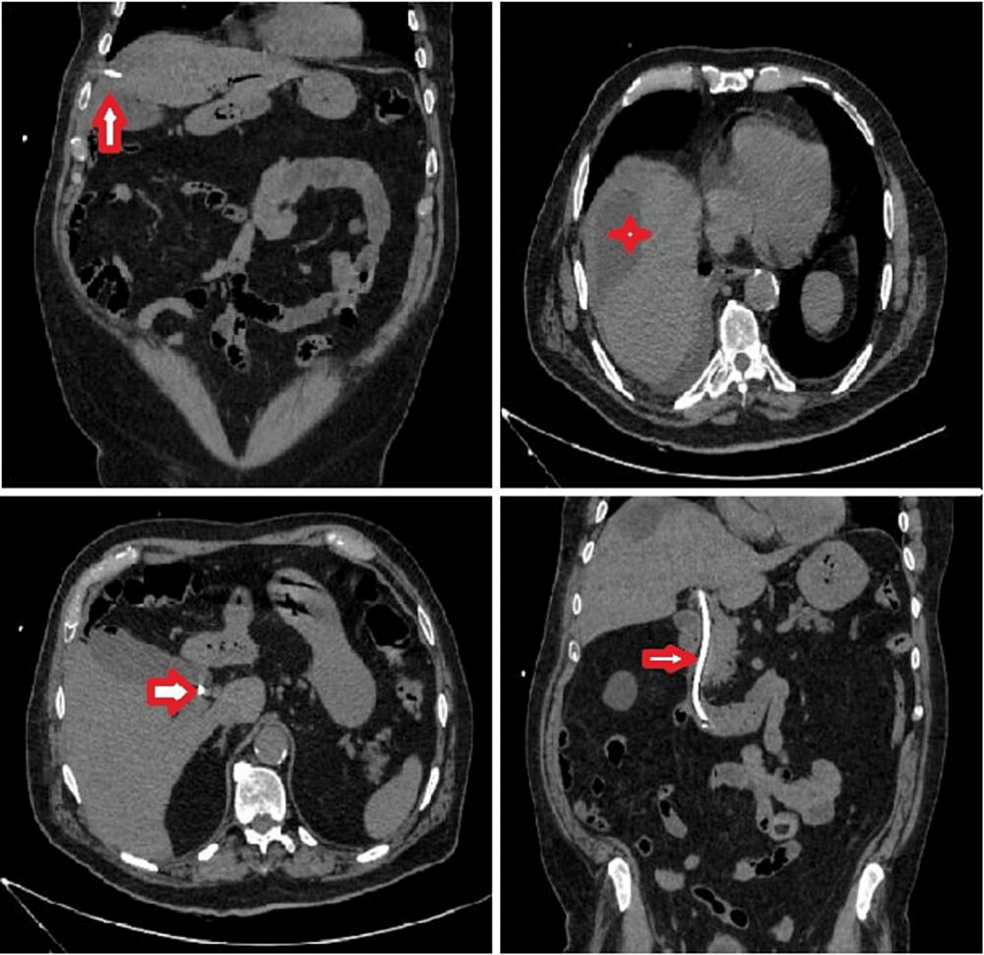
Images of the abdominal CT following percutaneous drainage of the collection and common bile duct stenting The white arrow points to the drain, the star represents the fluid collection smaller in size, and the arrow with blacked margins points to the stent within the common bile duct.
